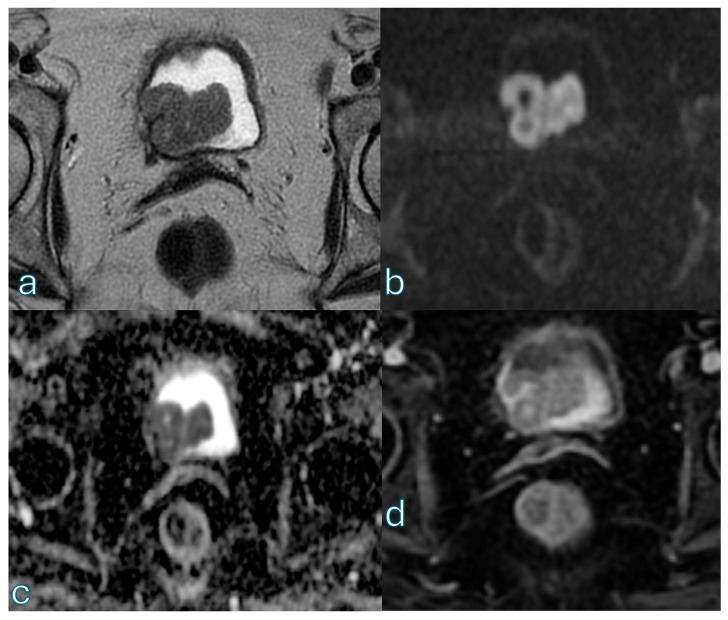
Axial T2-weighted imaging (T2WI) (a) demonstrates a pedunculated exophytic lesion arising from the right lateral bladder wall, measuring approximately 3 cm in maximum diameter, DCE provides temporal information on lesion, a feature that could suggest a higher VI-RADS category based on size alone. On diffusion-weighted imaging (DWI) (b), the lesion shows the characteristic inchworm sign, with curvilinear diffusion hyperintensity confined to the mucosal layer and corresponding signal on the ADC (c) map, already orienting the assessment toward a VI-RADS 2 category. DCE (d) imaging confirms preservation of the muscularis propria without evidence of early tumoral infiltration. Overall, despite its size, the combined imaging findings are consistent with a VI-RADS score of 2, highlighting the pivotal role of diffusion-weighted imaging in lesion risk stratification.
